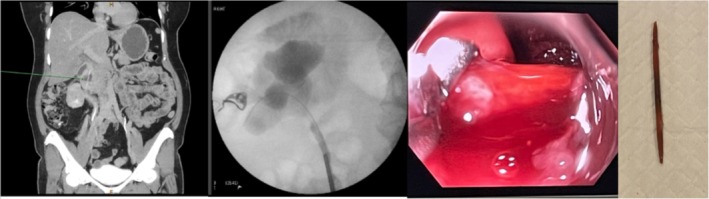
From left to right: (1) computed tomography intravenous pyelogram showing linear foreign body within gastro‐intestinal tract, (2) retrograde pyelogram showing contrast within the duodenum, (3) endoscopic retrieval of toothpick and (4) toothpick upon retrieval.
